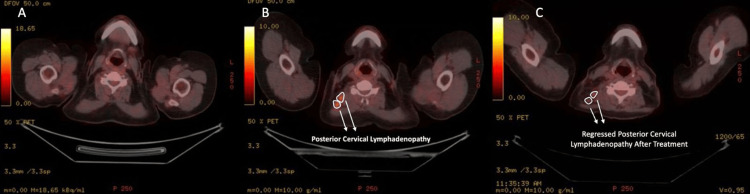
Serial axial 18F-FDG PET/CT findings demonstrating the development and regression of cervical lymph node metastasis A) Axial preoperative 18F-FDG PET/CT imaging showing no cervical lymphadenopathy B) Axial 18F-FDG PET/CT imaging performed 11 months postoperatively, demonstrating metastatic cervical lymphadenopathy (SUV value: 4.69) C) Axial 18F-FDG PET/CT imaging obtained 17 months postoperatively, after treatment, showing lymph nodes with dimensional and metabolic regression (SUV value: 2.44) 18F-FDG PET/CT: 18F-fluorodeoxyglucose positron emission tomography/computed tomography; SUV: standardized uptake value
